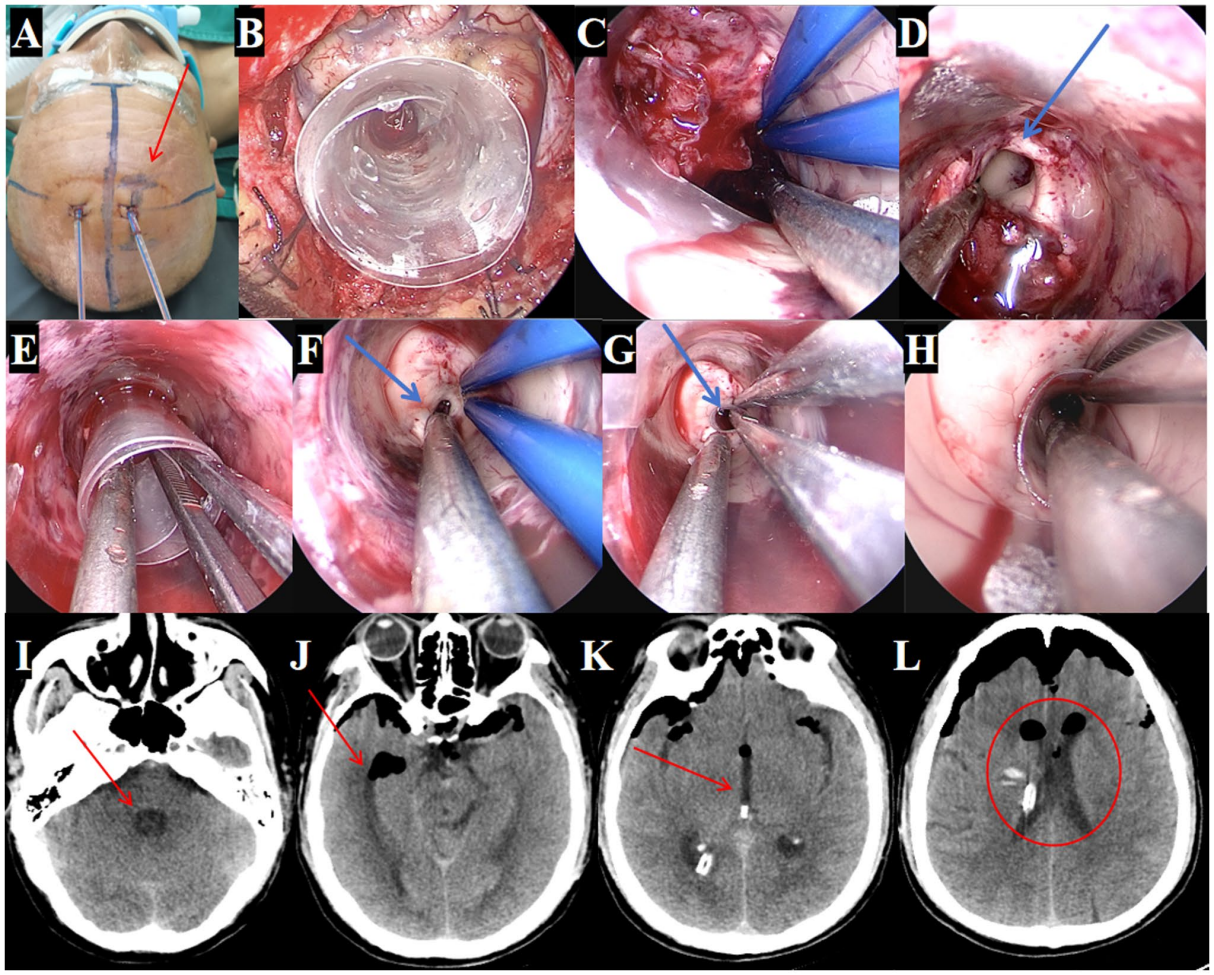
Surgical procedure using the TSNCT for treating patients with complete ventricular casting. (A) Selection of the existing right lateral ventricle frontal horn approach (→ arrow marks entry site). (B) Insertion of a outer sheath through the puncture pathway. (C) Removal of hematoma from the lateral ventricle. (D) Clearance of hematoma in the foramen of Monro region (→ arrow highlights cleared foramen). (E) Insertion of the middle sheath through the foramen of Monro. (F, G) Exploration of the midbrain aqueduct (→ arrow marks aqueduct lumen). (H) Insertion of a mini-sheath through the midbrain aqueduct and removal of hematoma from the fourth ventricle via the mini-sheath. (I–L). Postoperative CT indicating successful hematoma clearance (→ arrows mark evacuated regions; ◯ circles show restored ventricular patency).
